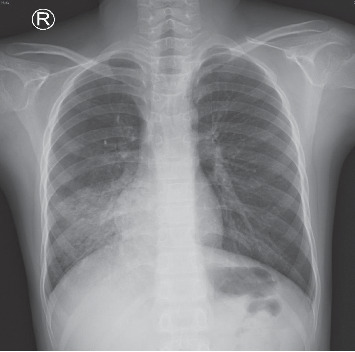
Chest X-ray documented right lower pneumonia.
